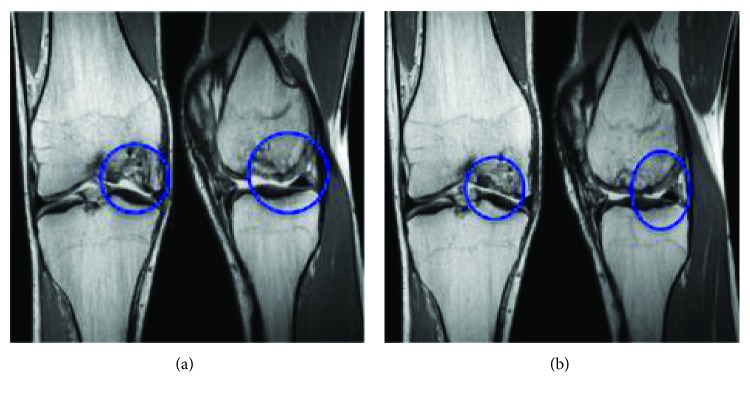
(a) Pretreatment proton density- (PD-) weighted coronal and sagittal MRI images of the knee showing the isolated chondral defect involving the central weight-bearing area of the medial femoral condyle. (b) Posttreatment PD-weighted coronal and sagittal MRI imaging at 18 months indicating articular cartilage regeneration at the site of the osteochondral defect [74].
